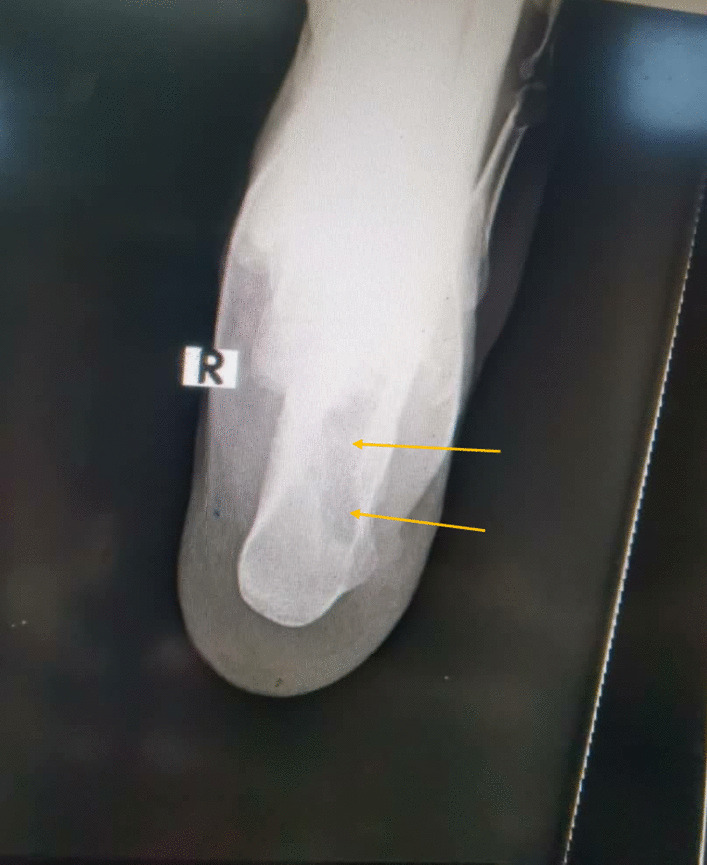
AP view X-ray revealed calcaneus scalloping lesion forming 2 cavities posterior to the posterior facet of the calcaneus consistent with chronic osteomyelitis
